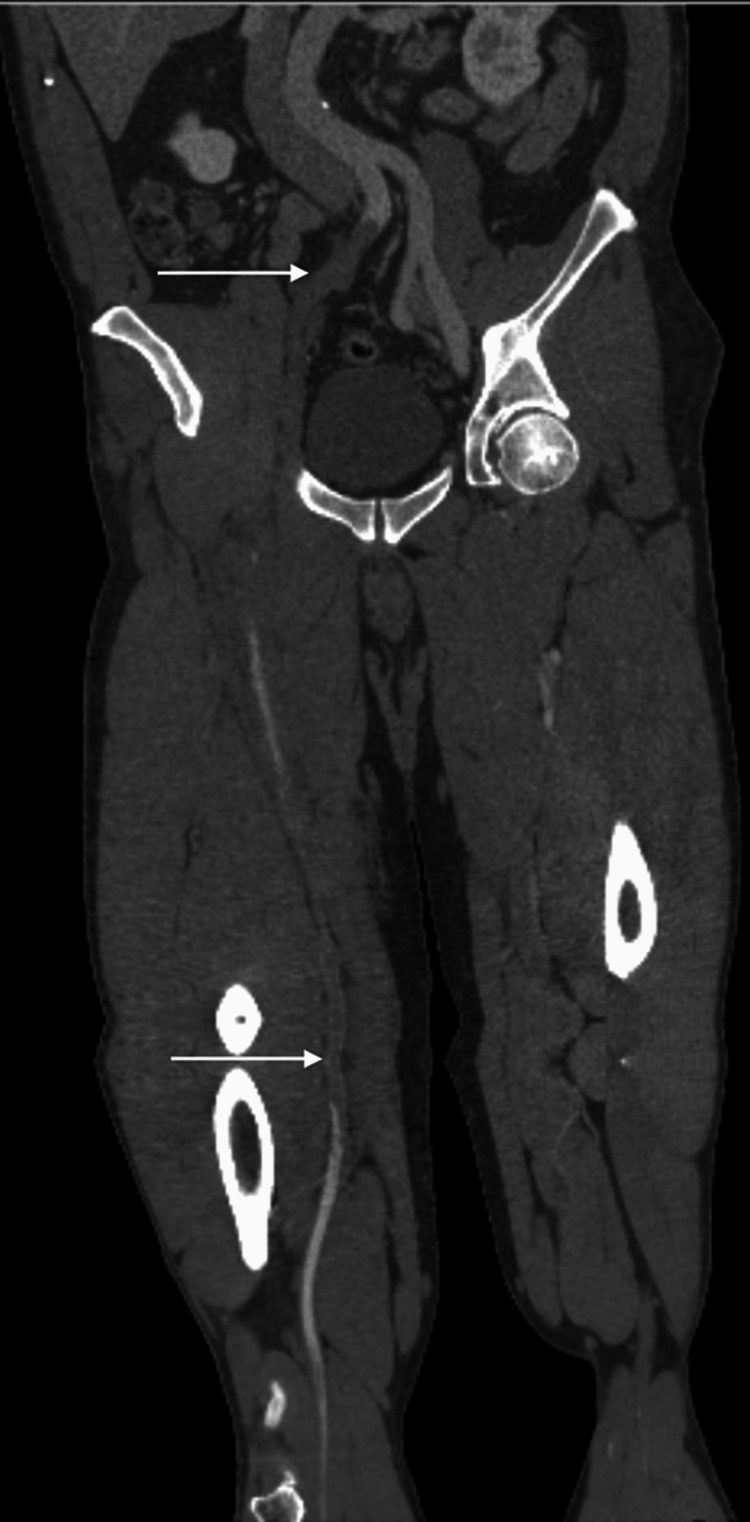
Computed tomography angiography demonstrating thrombus of the right iliac and femoral arteries
